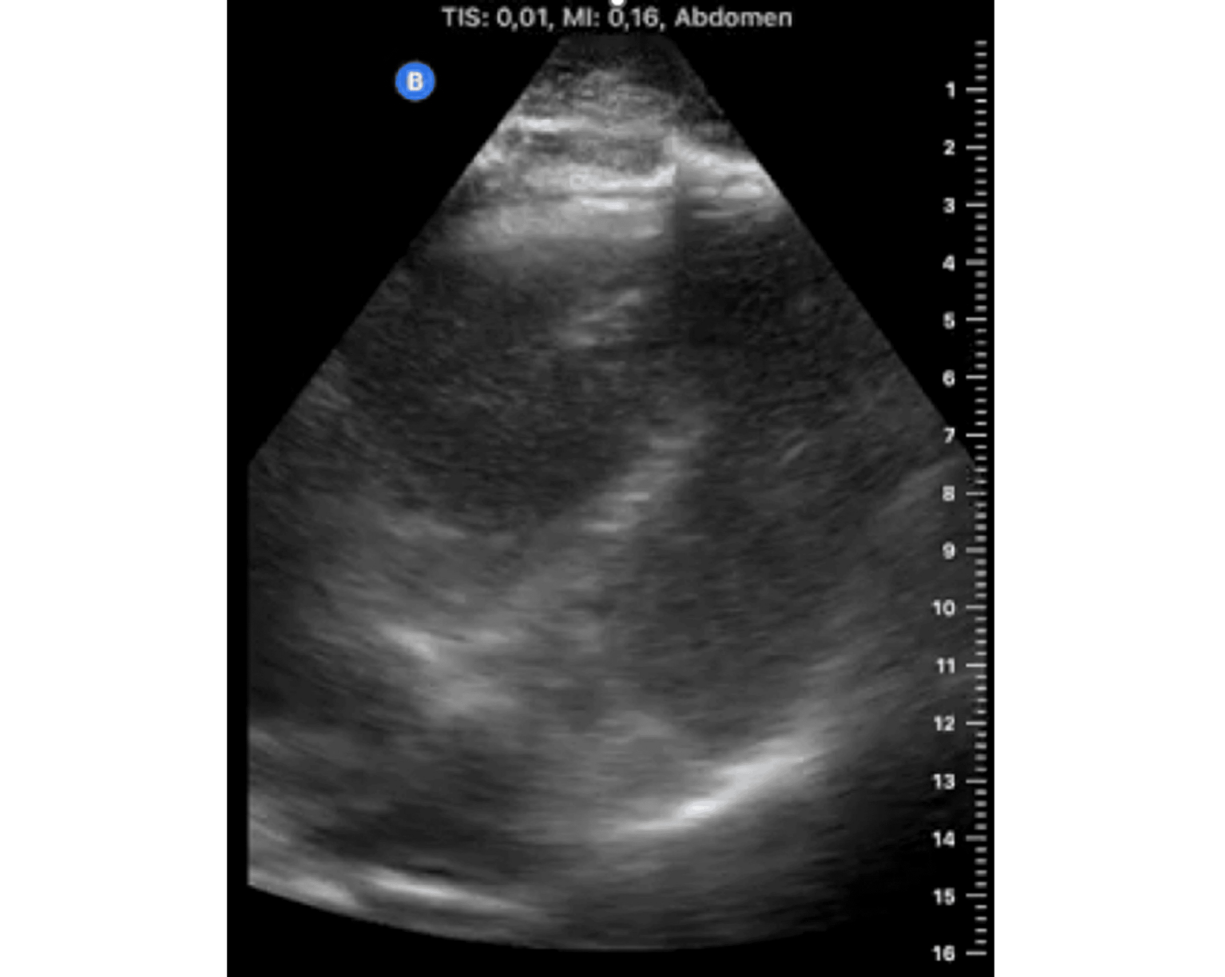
Focused cardiac ultrasonography on scene. Focused cardiac ultrasound performed by the emergency medical services physician revealed signs of severe right ventricular strain, consistent with acute pulmonary embolism. The right ventricle appeared dilated and hypokinetic, while the left ventricle was small and hyperdynamic.
